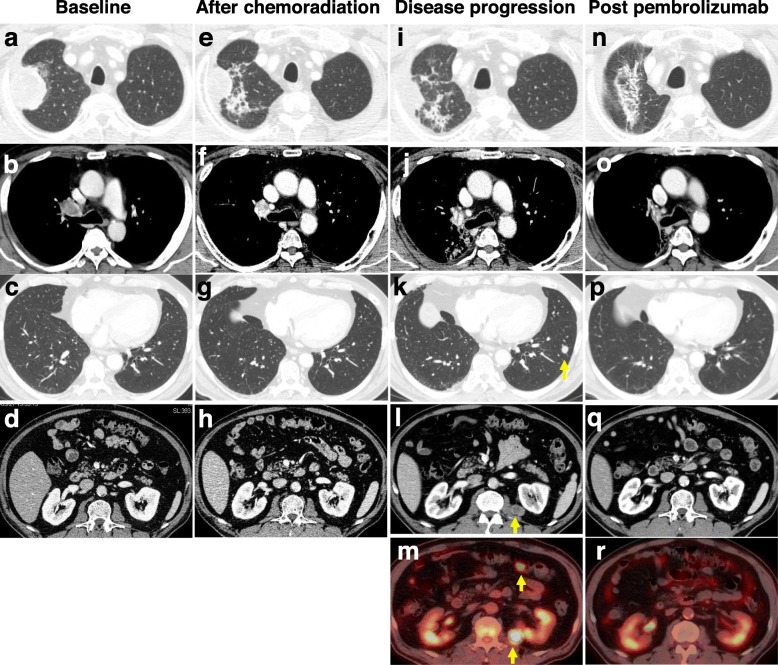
Chest computed tomography (CT) and 18F-fluorodeoxyglucose (FDG) positron emission tomography (PET)-CT. Imaging findings during the patient’s course (a, b, c, and d) at baseline (before chemotherapy), (e, f, g, and h) after chemoradiotherapy and before durvalumab consolidation therapy, (i, j, k, l, and m) after the seventh round of durvalumab, and (n, o, p, q, and r) after the ten cycles of pembrolizumab. a and b CT showing right upper lobe and hilum involvement at the time of diagnosis (May 2018). e and f CT showing the response to chemoradiotherapy (August 2018). k and l CT showing progressive disease during durvalumab therapy (November 2018). New metastatic nodules were visible in the left lower lobe (k, *arrow*) and the left psoas (l, *arrow*). m PET-CT showed FDG accumulation in the left psoas and the abdominal lymph node (*arrows*) (November 2018). n, o, p, q, and r Both primary and metastatic lesions were dramatically improved by pembrolizumab treatment
